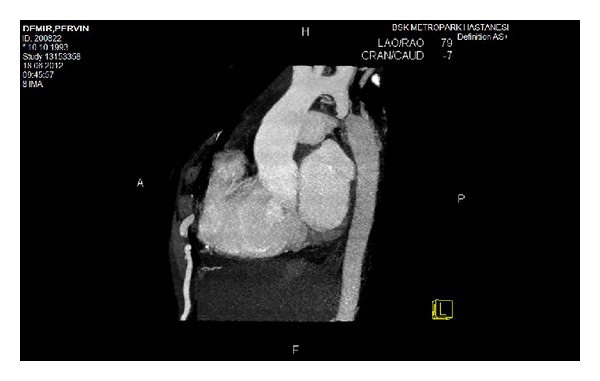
Sinus of Valsalva aneurysm, coarctation of aorta, and dilated internal thoracic artery in CT angiography.
